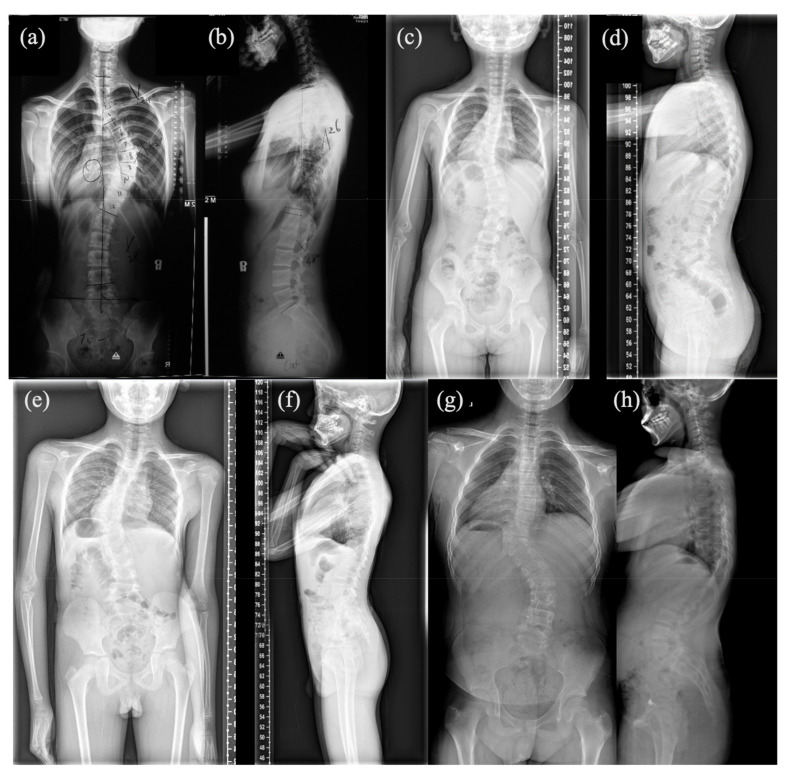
Anteroposterior and lateral spinal X-ray of four patients: (a,b) Anteroposterior and lateral spinal X-ray of patient #1; (c,d) Anteroposterior and lateral spinal X-ray of patient #2; (e,f) Anteroposterior and lateral spinal X-ray of patient #3; (g,h) Anteroposterior and lateral spinal X-ray of patient #4.
